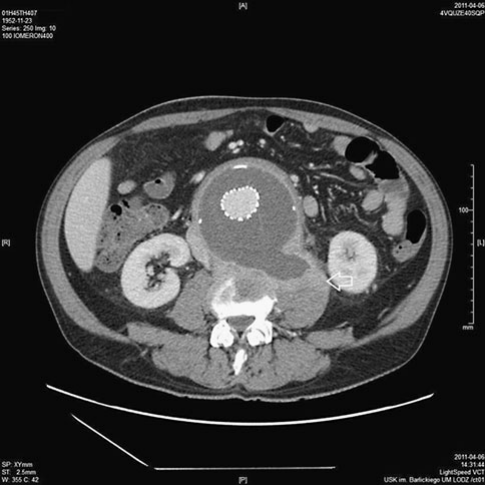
Angio-CT axial scan of aneurysm below renal arteries after EVAR. Soft tissue infiltration with postcontrast enhancement is visible, which encompasses the aorta, the inferior vena cava, and the hematoma in the left iliopsoas muscle (*arrow*). Osteolysis of the L4 vertebral body is evident
